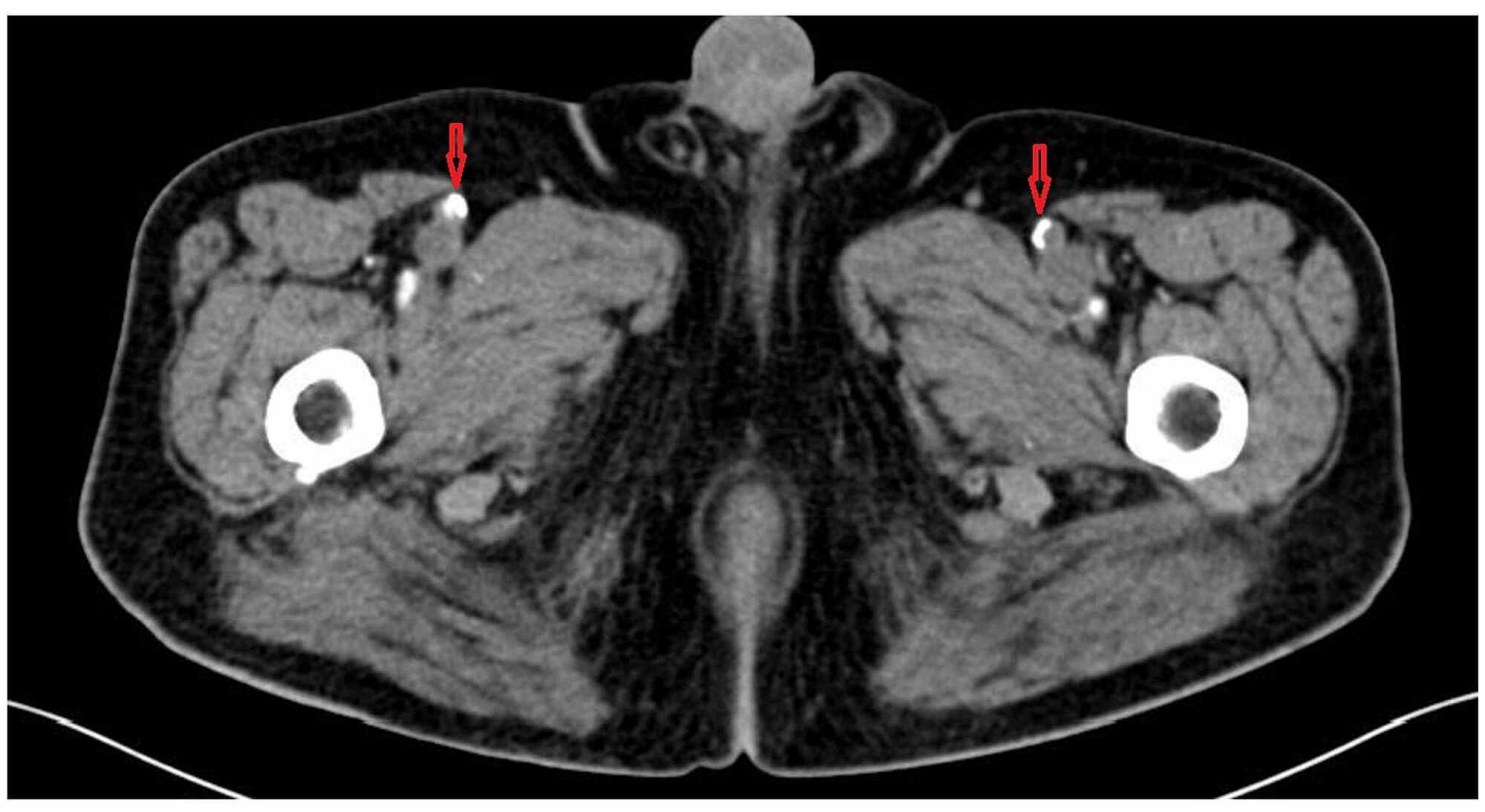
Computed tomography angiogram (CTA) of lower extremities showing acute vascular occlusion at the level of left superficial femoral artery (red arrow).
